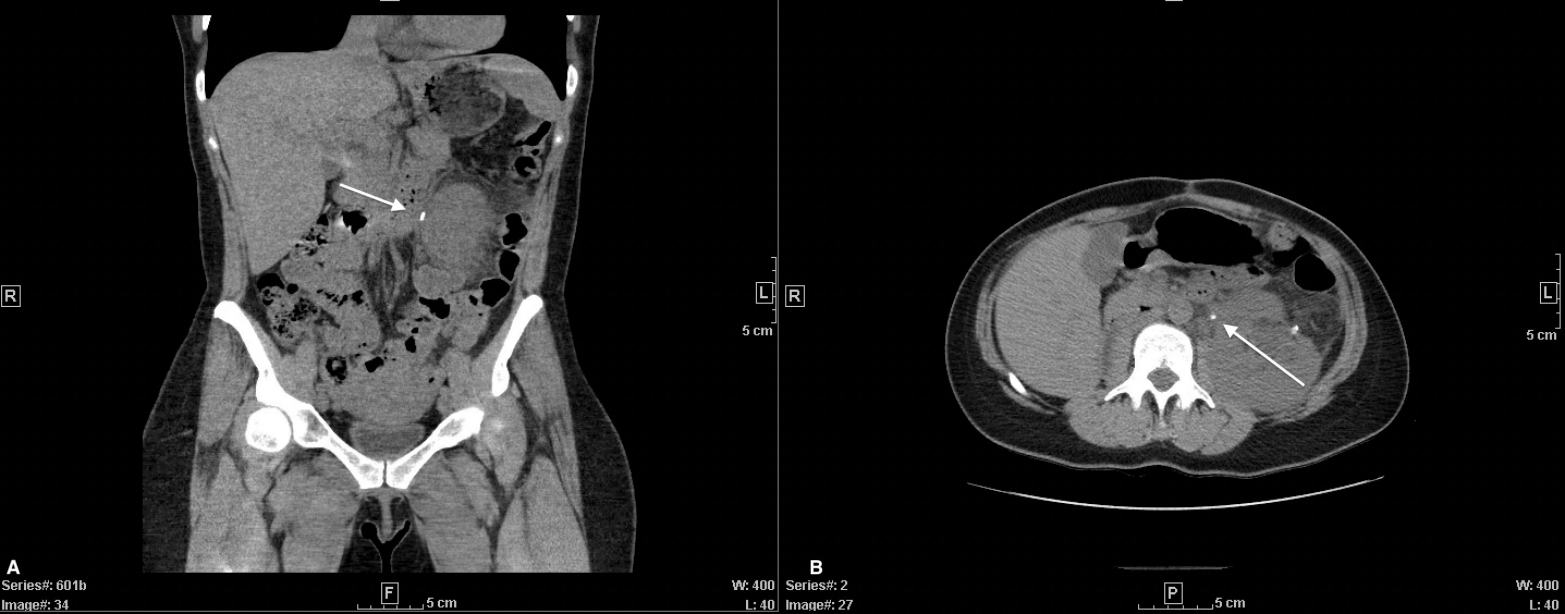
CT-Abdomen without contrast. Coronal (A) and axial (B) views displaying left-sided obstructing UPJ stone.
